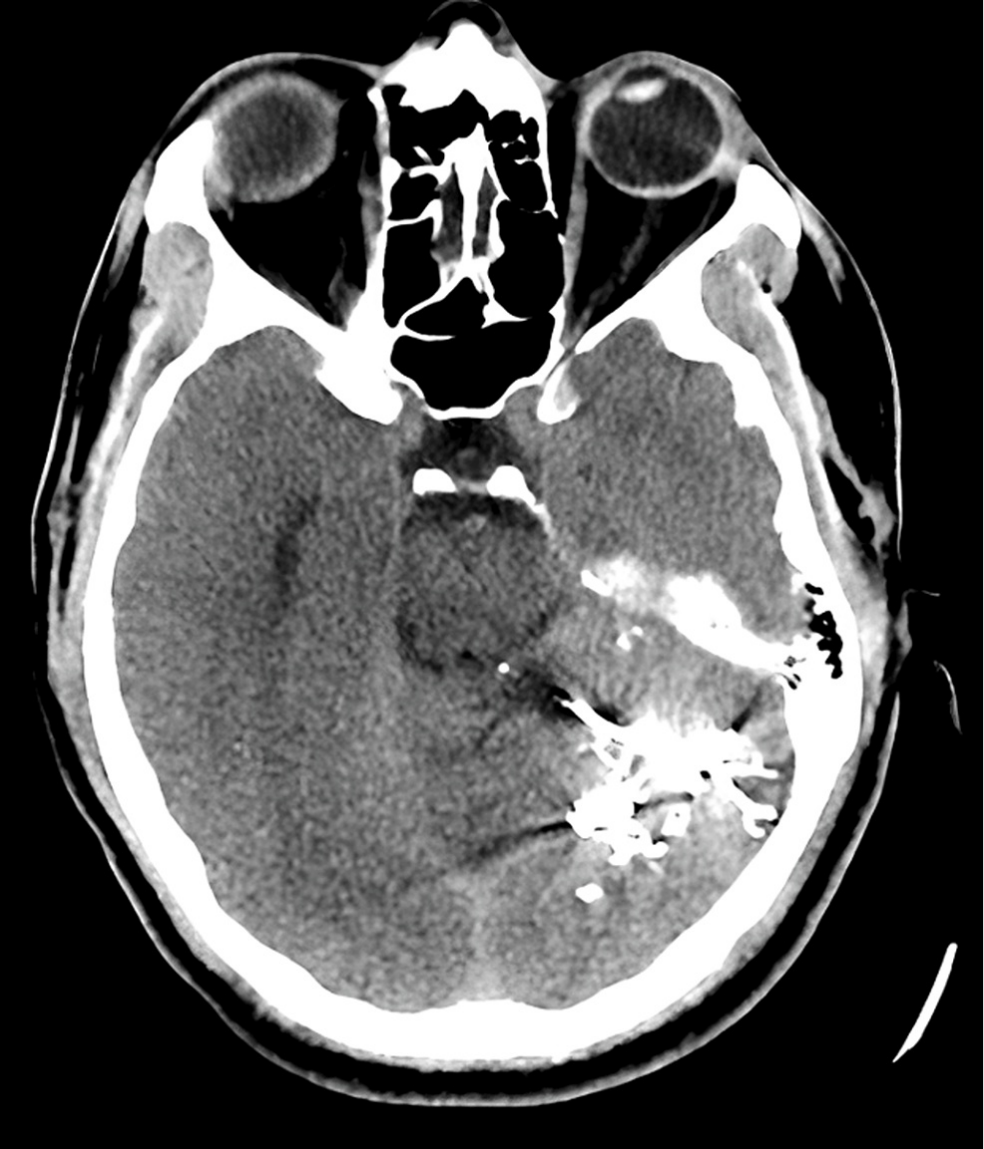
Post-embolization CT brain without contrast
